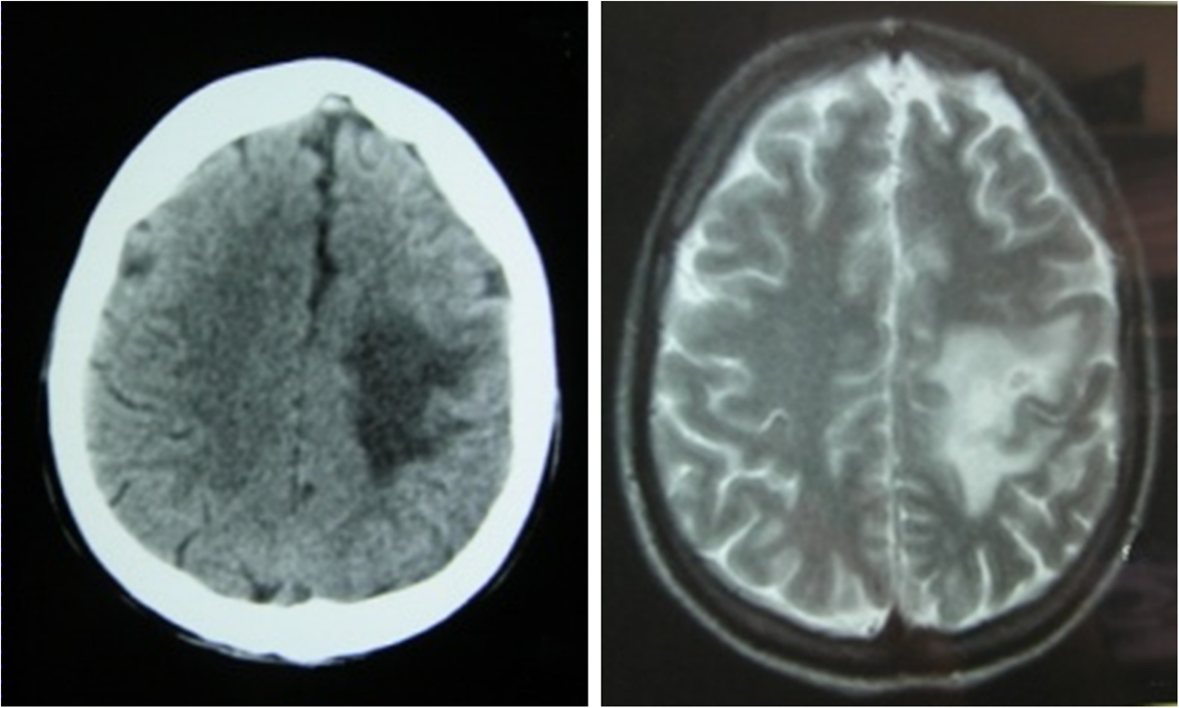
Cerebral MRI showing enhanced lesion in the left lobe.
